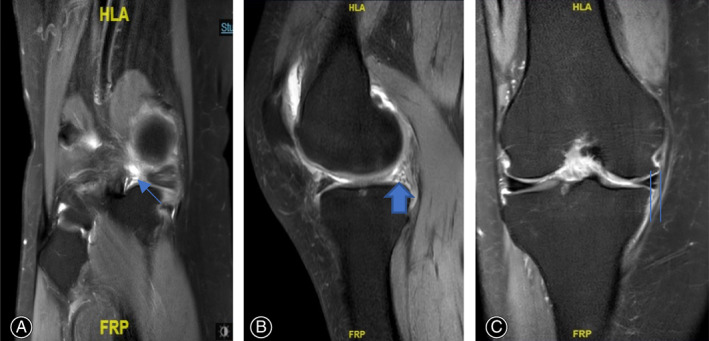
Thirty‐one months later after arthroscopic non‐anatomical repair of MMPRT to PCL of a 67‐year‐old woman patient with right knee MMPRT and normal limb alignment. Postoperative MRI images indicated almost complete healing of MMPRT. (A) MMPRT’s almost complete healing indicated by long fine arrow in T2 coronal view. (B) Almost triangular shape of MMPR indicated by long‐coarse arrow. (C) Decreased medial meniscus extrusion degree indicated by two lines.
